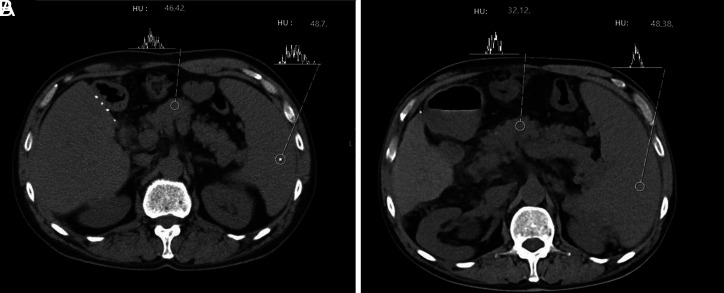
(A) Non-contrast CT image of the same patient in figure 2 shows attenuation values of pancreas (P) as 46 HU and spleen (S) as 48 HU. P-S difference: -2; P/S ratio: 0.96. (B) Control non-contrast CT after acute pancreatitis shows the same values as 32 HU and 48 HU respectively. P-S difference: -16; P/S ratio: 0.67. This ratio is compatible with fatty pancreas.
